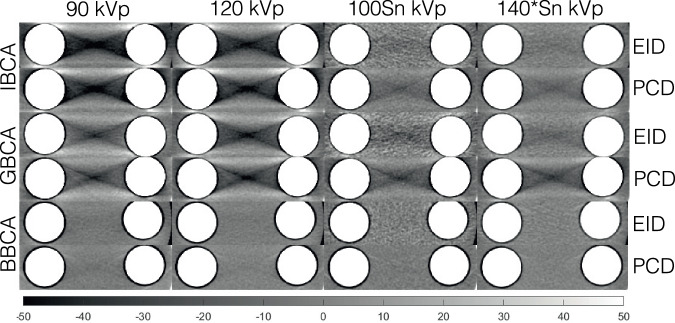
Cropped samples of all images used in this study showing the shadow BH artifacts for different CAs at different x-ray tube settings. Iodine/bone BH correction was intentionally turned off to accentuate the artifacts. BBCA, Bismuth-based contrast agent; EID, Energy-integrating detector; GBCA, Gadolinium-based contrast agent; IBCA, Iodine-based contrast agent; PCD, Photon-counting detector
